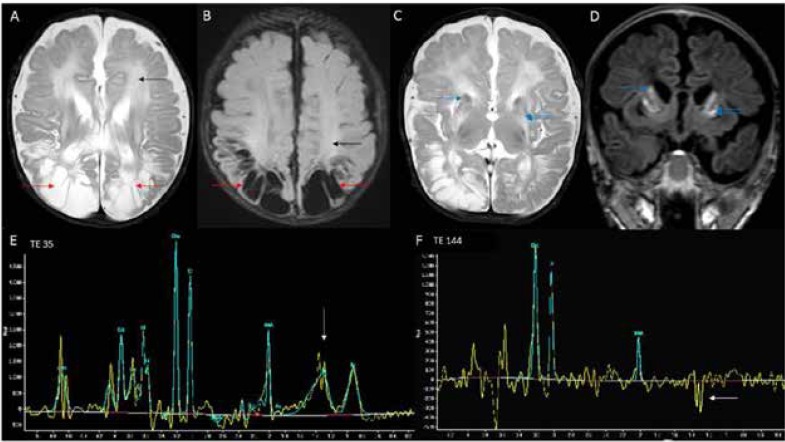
MRI brain T2 axial (A) and FLAIR (B) images show bilateral parieto-occipital cystic encephalomalacia (red arrows) and deep white matter hyperintensities (black arrows). Volume loss and signal changes of caudate (blue arrow- single) and putamen (blue arrows- double) are seen in T2 axial (C) image. Corresponding T1 coronal image (D) shows hyperintense signal (blue arrows- single and double) which could represent early calcification. Also note the sparing of thalamus. MRS from parenchyma shows peak at TE 35 (E) with inversion at TE 144 (F) suggestive of lactate
